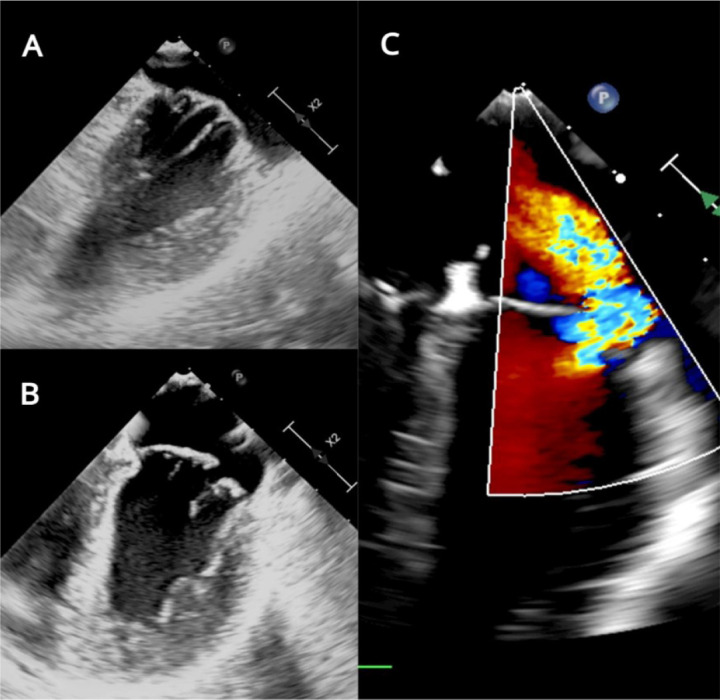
Transesophageal echocardiogram, two-dimensional view of mitral valve.
